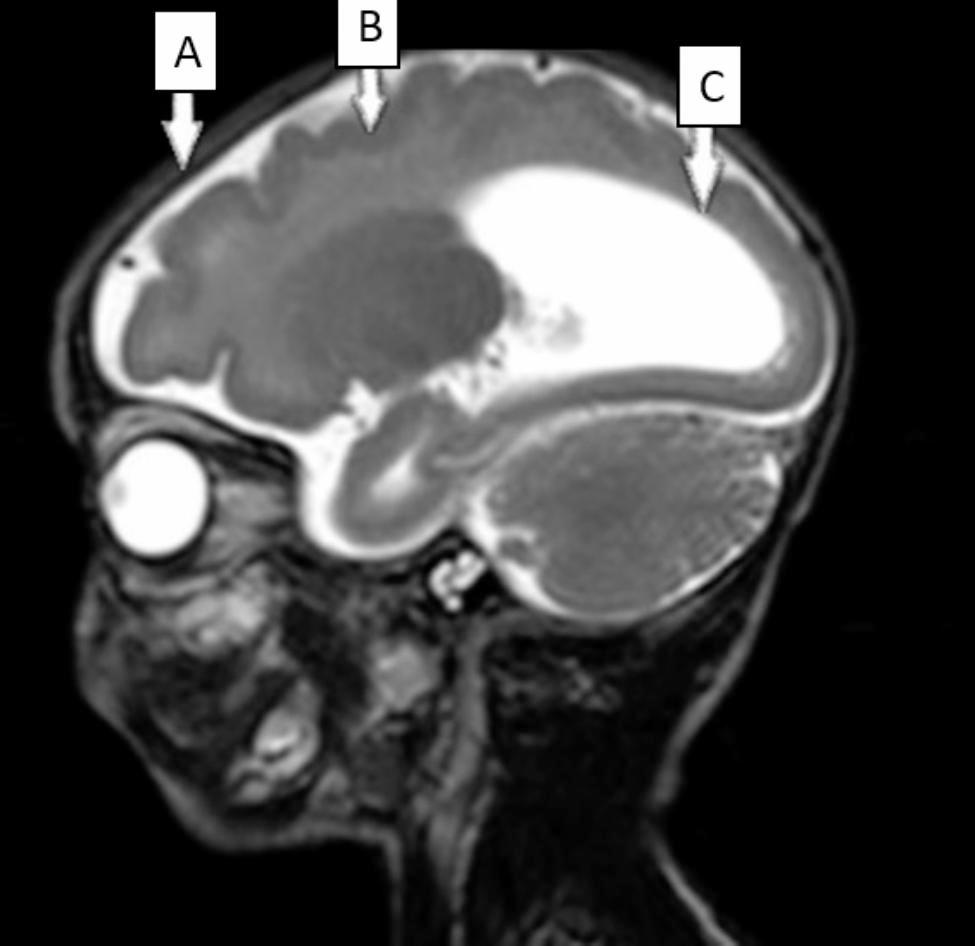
MRI results in our patient showing (A) superior frontoparietal lissencephaly, (B) subcortical band heterotopia (double-cortex), (C) enlargement of occipitotemporal lateral ventricles
